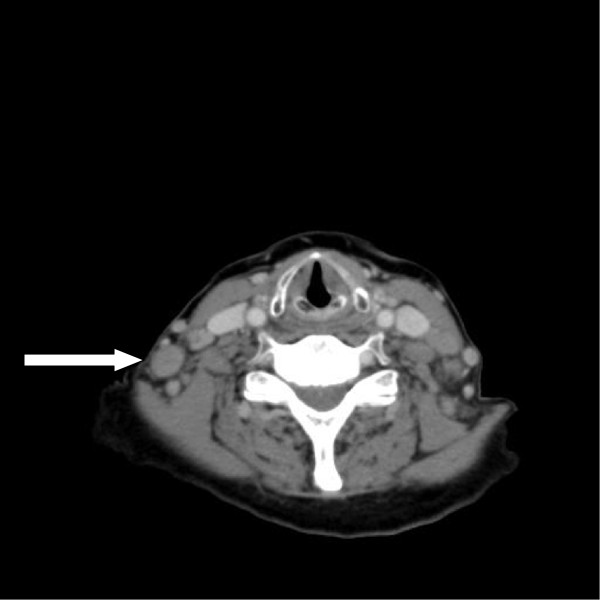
CT scan of the neck before starting belinostat therapy (January 2010) showing multiple lymph nodes mainly at the right site of the neck (arrow).
